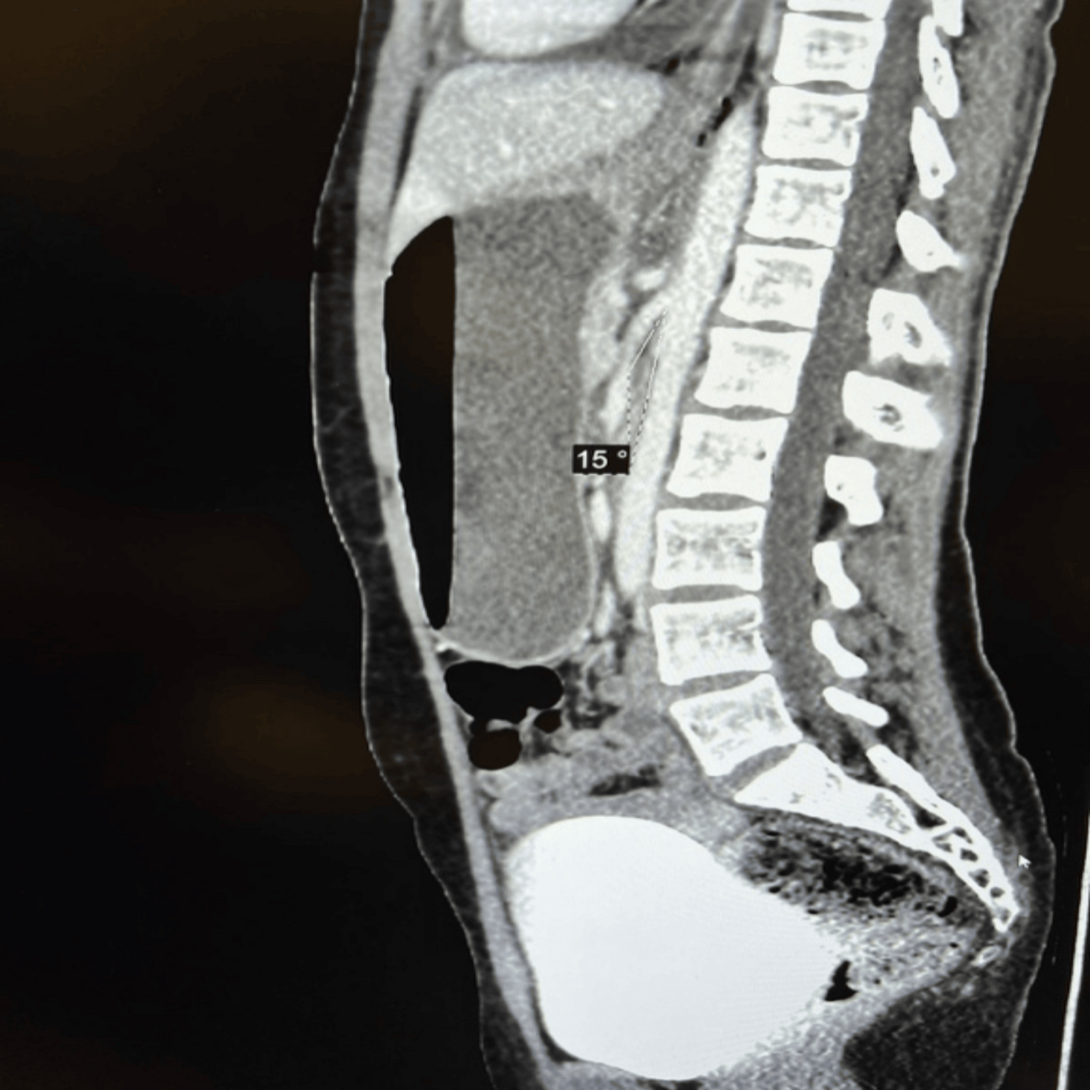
CT sagittal section demonstrating an aortomesenteric angle of 15°. CT: computed tomography.
